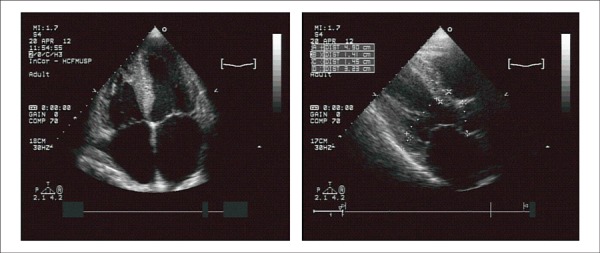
Echocardiogram - a) Four-chamber view: marked enlargement of the left and right
